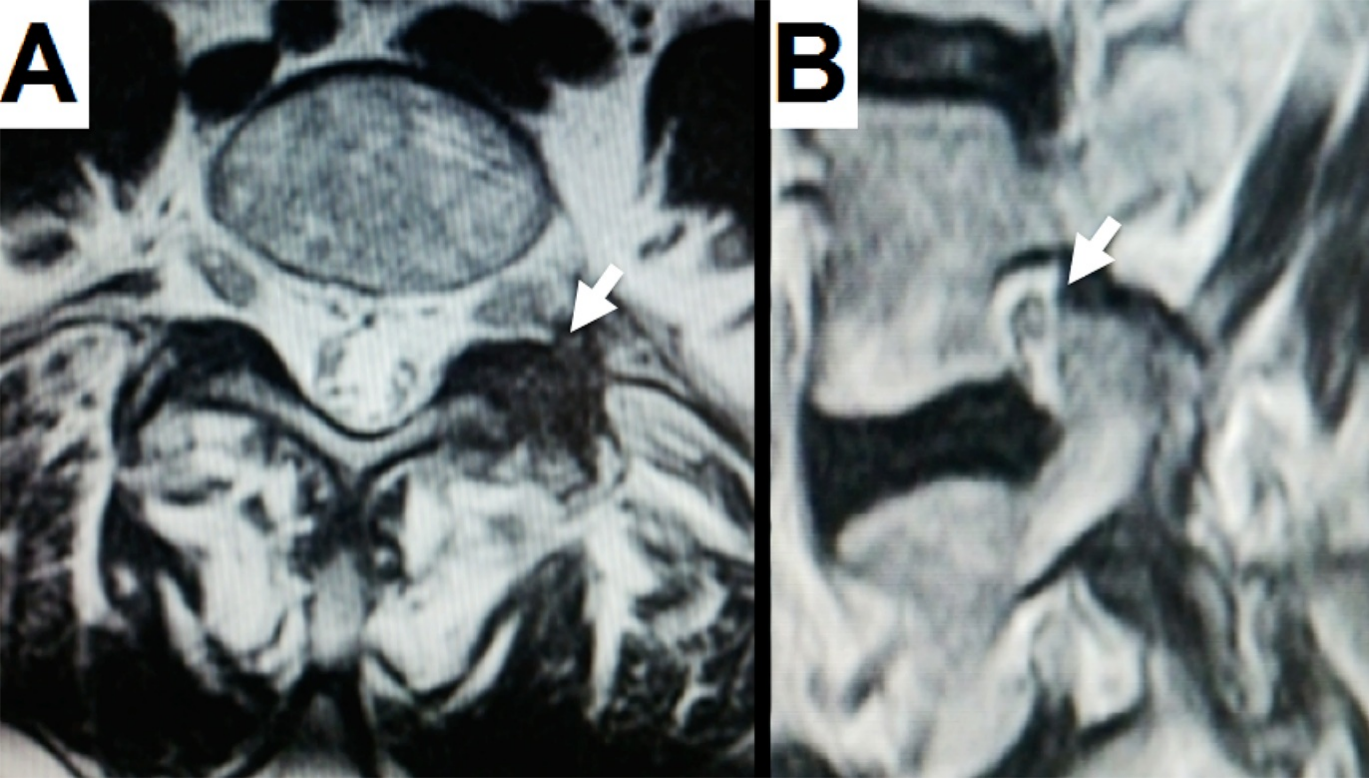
Postoperative MRI A: Axial T2 shows that the cyst has been completely removed (white arrow) and the lateral facet joint has been only minimally drilled off. B: Sagittal T2 indicates no scar tissue from the cyst behind the facet joint (white arrow).
